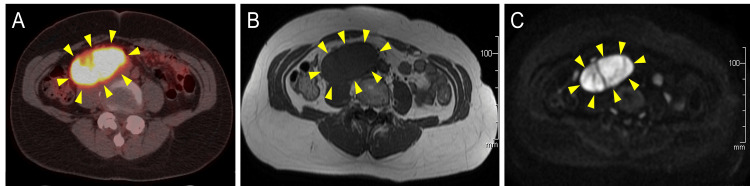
Positron emission tomography (PET) and magnetic resonance imaging (MRI) showing a retroperitoneal tumor (A) PET showed the mass with a maximum standardized uptake value of 21 (arrowheads). (B) MRI showed an isointense signal on T2-weighted images (arrowheads). (C) MRI showed high signal on the diffusion-weighted image (arrowheads).
